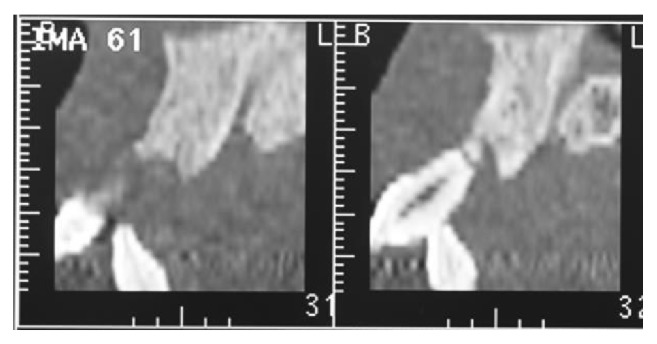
CT scans of patient upon presentation showing resorption of buccal and palatal bone adjacent to maxillary incisors.
